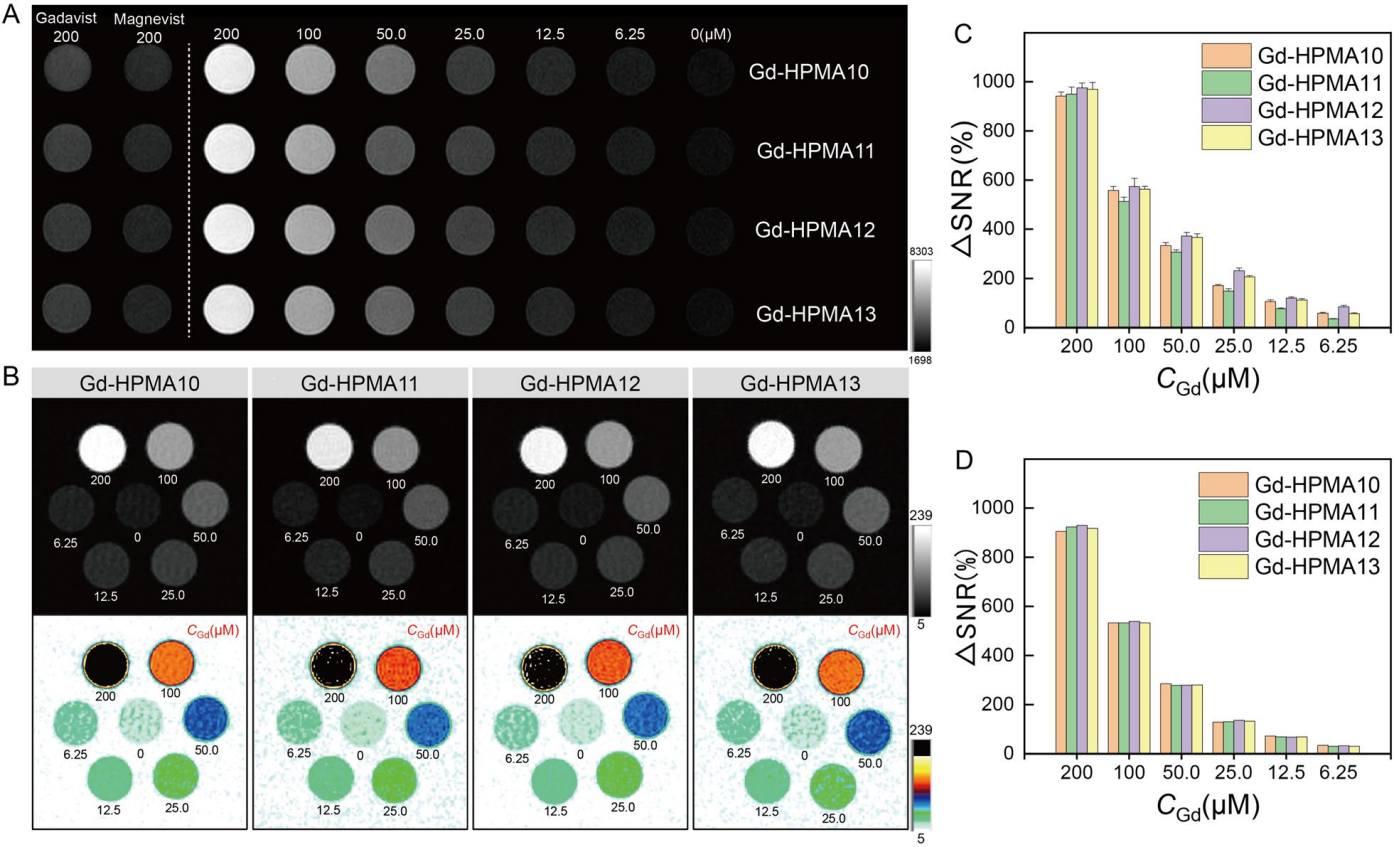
T*_1_-weighted MR imaging of Gd-HPMA macrochelates at various concentrations. A: *T*_1_-weighted MR images of Gd-HPMA macrochelates with various *C*_Gd_ (0 ~ 200 μM) compared with the commercial Gadavist^®^ and Magnevist^®^ (200 μM) observed by a 3.0 T clinical MRI system. B: The black and white and corresponding pseudo-color images of *T*_1_-weighted MR images for Gd-HPMA macrochelates observed by a 7.0 T MRI scanner. C, D: ΔSNR of the MR images A, B for Gd-HPMA macrochelates with various *C*_Gd_ observed at 3.0 C or 7.0 T D
